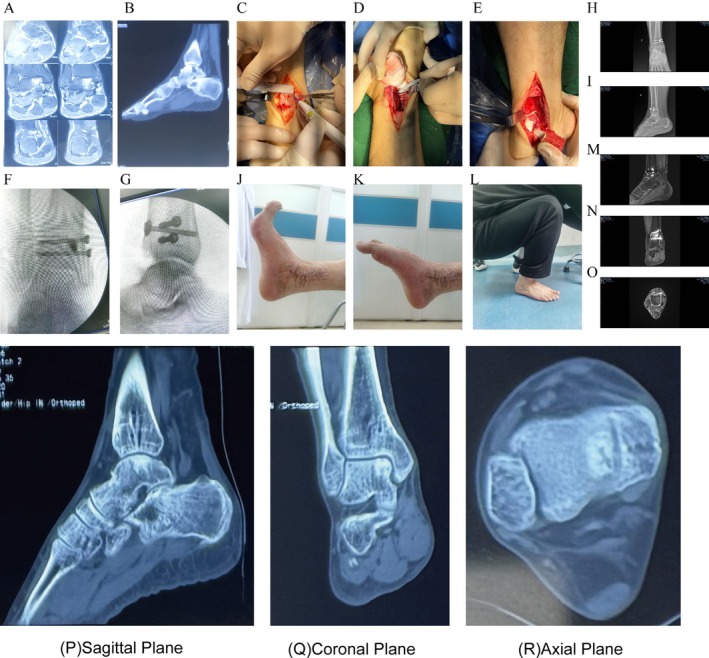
Images from a typical case of a large talar cyst. (A) Preoperative magnetic resonance image (MRI); (B) preoperative computed tomography (CT) image; (C–E) intraoperative surgical images, measurement of osteotomy range (C), triplanar osteotomy of the medial ankle (D), revealing and clearing the talus cyst (E); (F and G) intraoperative perspectives of the right ankle joint; (H and I) reexamination of the right ankle arthrosis 3 months after surgery; (J–L) re‐examination of ankle dorsiflexion, flexion function, and activity of ankle joint in squatting position with weight bearing 6 months after surgery; (M and N) MRI of the right ankle 15 months after surgery. Sagittal view (M), coronal view (N), all the MRI levels of the right ankle joints showed that the talus cyst was well repaired, and there was no obvious inflammatory reaction around it (O); Postoperative CT follow‐up data (P–R) at 30 months, it can be observed that the grafted bone has achieved complete fusion.
